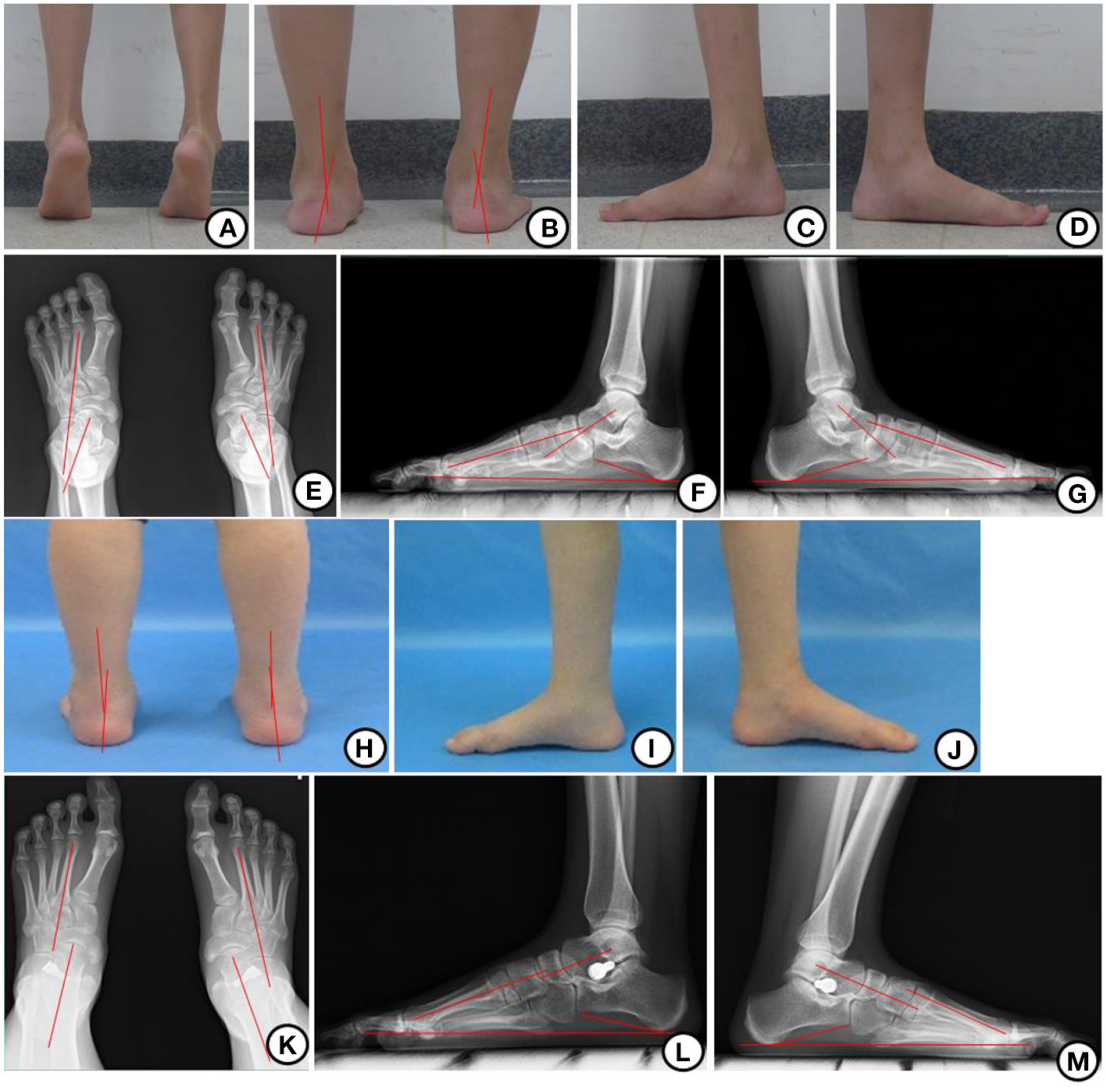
Appearance and x-ray before and after operation. (A) Hindfoot valgus could be corrected when calf raising before operation. (B) Hindfoot valgus before operation. (C, D) The medial appearance of left and right foot in one patient before the operation. (E) AP x-ray before operation showing T2MT angle. (F, G) Lateral x-ray before the operation showing Meary's and Pitch angle. (H) Hindfoot alignment was corrected 1 year after the operation. (I, J) The medial appearance of the left and right foot in one patient 1 year after the operation. (K) AP x-ray 1 year after the operation showing T2MT angle. (L, M) Lateral x-ray 1 year after the operation showing Meary's and Pitch angle.
